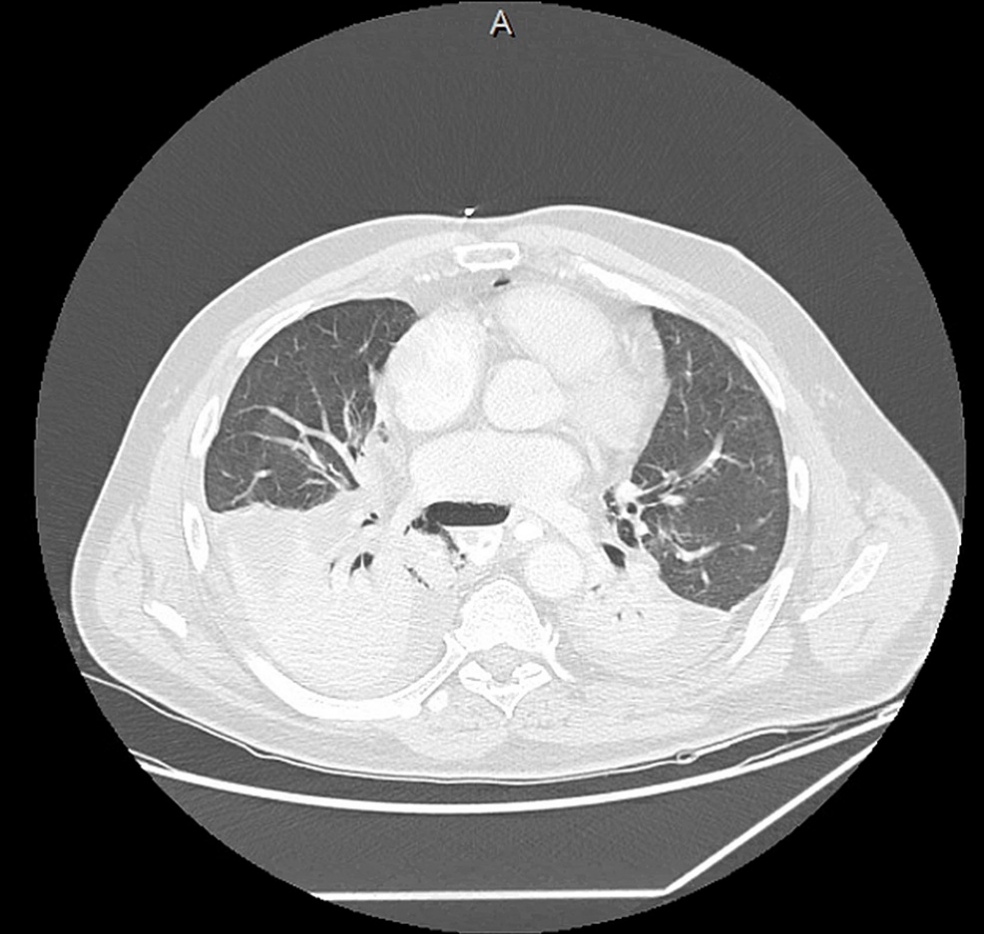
CT chest demonstrating extravasation of oral contrast from esophagus into the right mediastinum/hemithorax. Also note pneumomediastinum, bilateral pleural effusions, and consolidations
